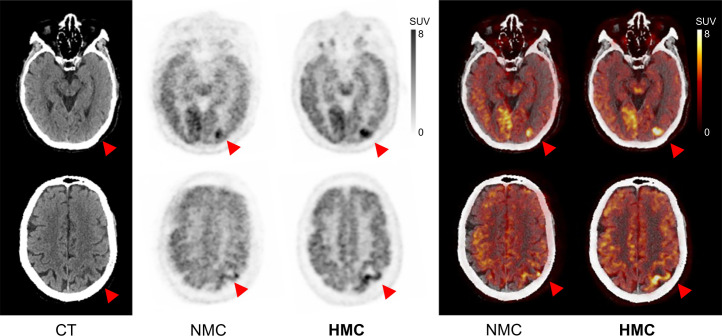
CT, PET, and PET/CT with HM before and after HMC in case of angioimmunoblastic T-cell lymphoma with suspected cerebral infiltration due to acute onset of neurologic symptoms. HM blur and misregistration were corrected after HMC. Areas of annular hypermetabolism with central hypometabolism in left parietal lobe (arrowheads) were revealed after HMC. Injected dose was 251.6 MBq, postinjection time was 90 min, frame duration was 3 min at single bed position, and body weight was 65 kg. Averaged and maximal HM distance of frontal lobe was 10.9 and 18.8 mm, respectively.
